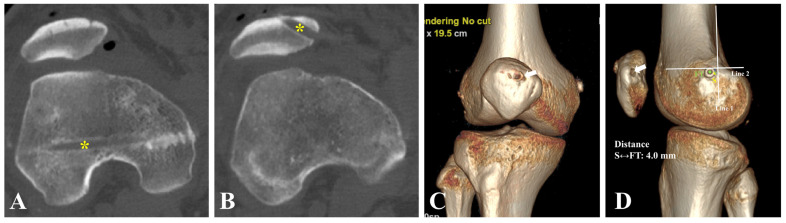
Postoperative imaging evaluation. Right knee. (A,B) Axial CT showing the femoral tunnel and the patellar tunnel (yellow asterisks). (C) Anterior view of three-dimensional CT reconstruction showing the patellar tunnel (white arrow). (D) Medial view of three-dimensional CT reconstruction. The patellar tunnel (white arrow) is located at the upper-third point of the medial patellar margin. The center of the arthroscopically localized femoral tunnel (green spot) lies 4.0 mm anterior to Schöttle’s point (yellow spot), which can be defined as precise tunnel placement according to prior studies [7,12,24]. CT, computed tomography. FT, femoral tunnel. S, Schöttle’s point.
